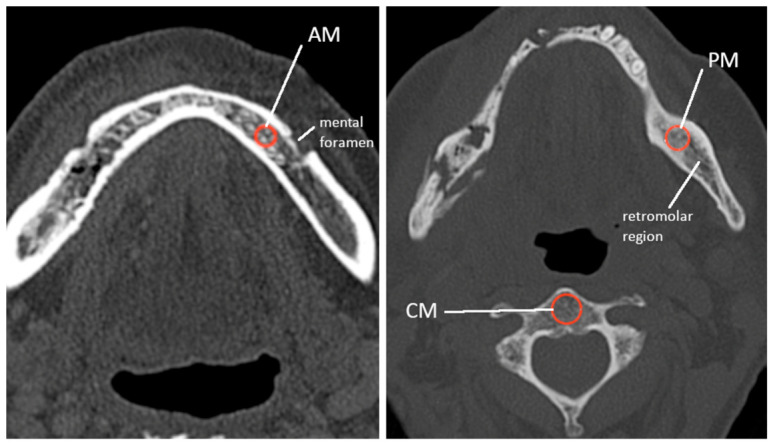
Locations of CT bone density measurements in the mandible and the 2nd vertebra. AM: anterior mandible; PM: posterior mandible; CM: control.
